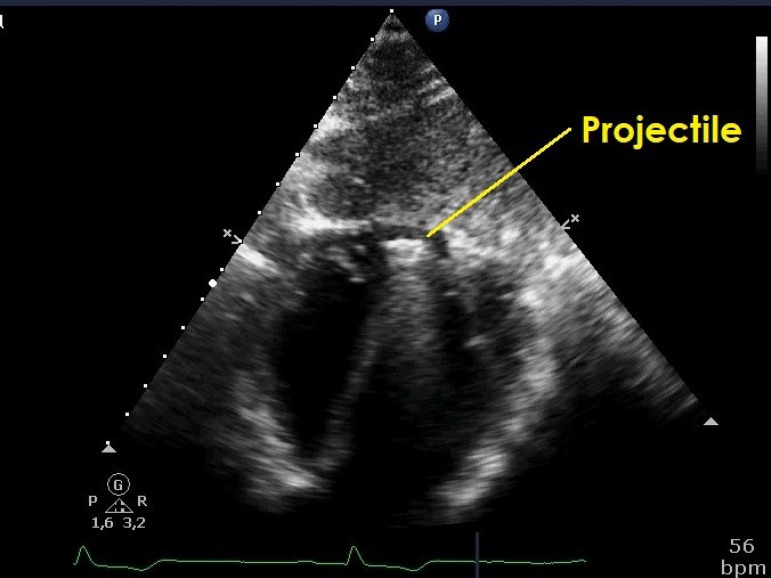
Echocardiogram showing the projectile inside the right ventricle.
